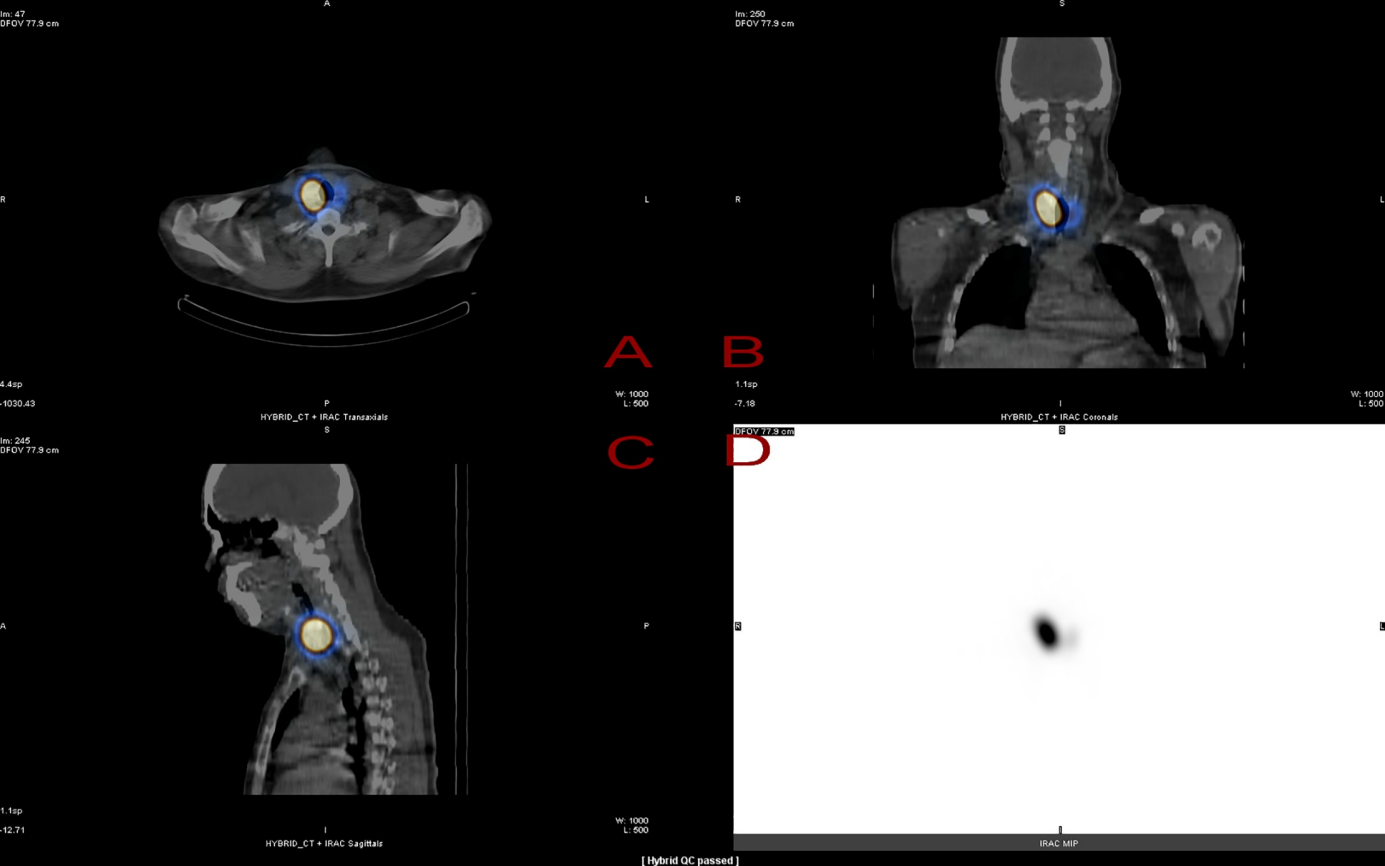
The SPECT/CT fusion plot of the residual thyroid gland for patient No. 1 is displayed as (A) axial view, (B) coronal view, (C) sagittal view of SPECT/CT fusion plot, and (D) maximum intensity projection (MIP), corresponding to the iterative reconstruction with the attenuation correction (IRAC) of the residual thyroid gland.
